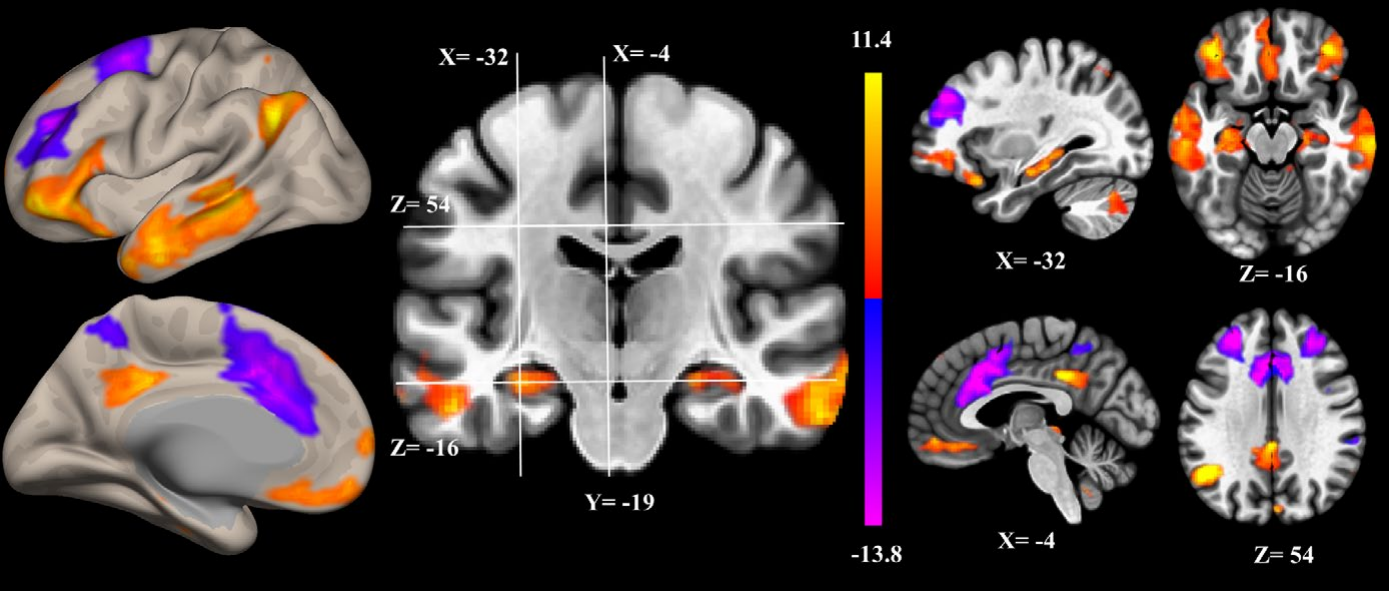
Z‐stat maps of independent component analysis of resting stage‐functional magnetic resonance imaging‐based covariant spatial patterns for default mode network
